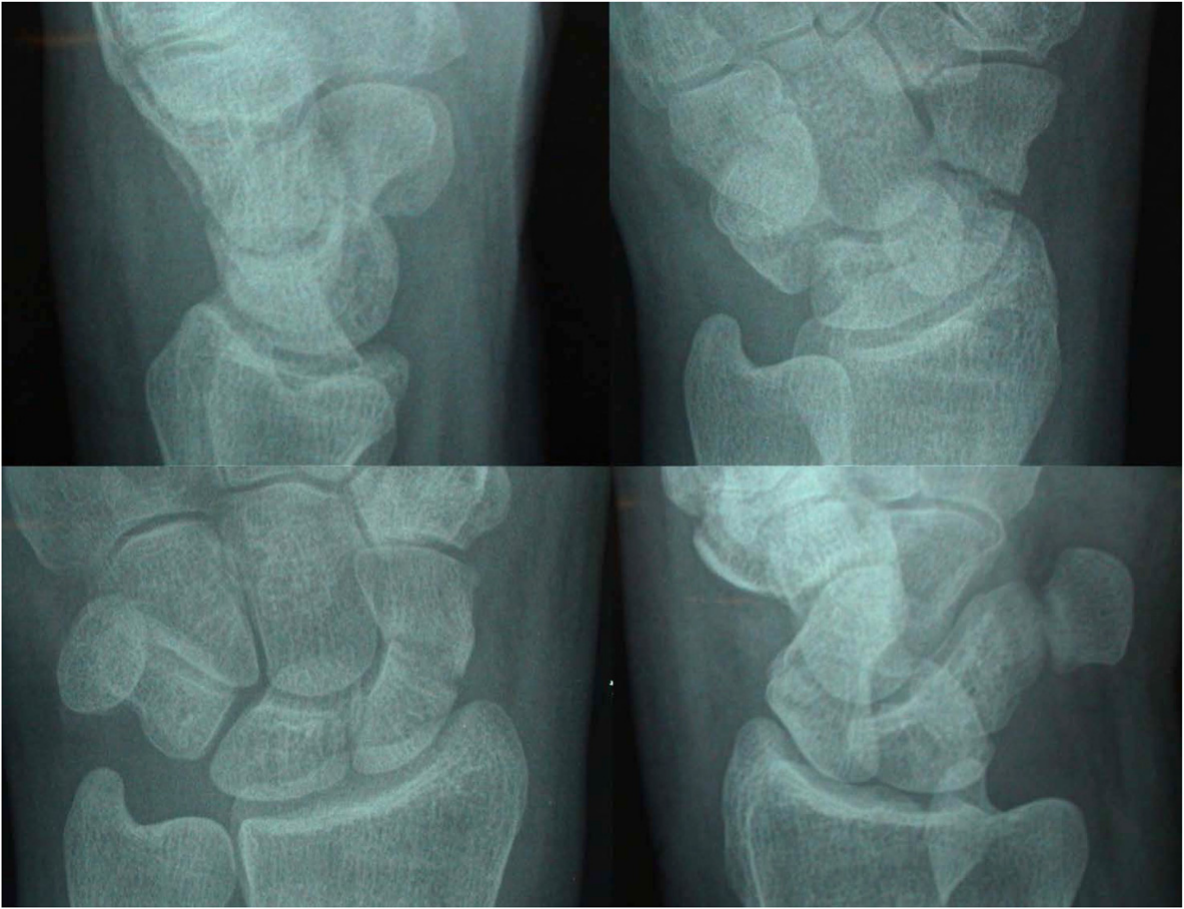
Radiographic views of a scaphoid fracture. Four radiographic views shown here, and a fifth elongated scaphoid view, establish the presence of a “clear” and ”bicortical” fracture of the waist of the scaphoid. Such patients are eligible for the SWIFFT trial. These radiographs also help determine whether the fracture is displaced for blocked randomisation after obtaining consent
